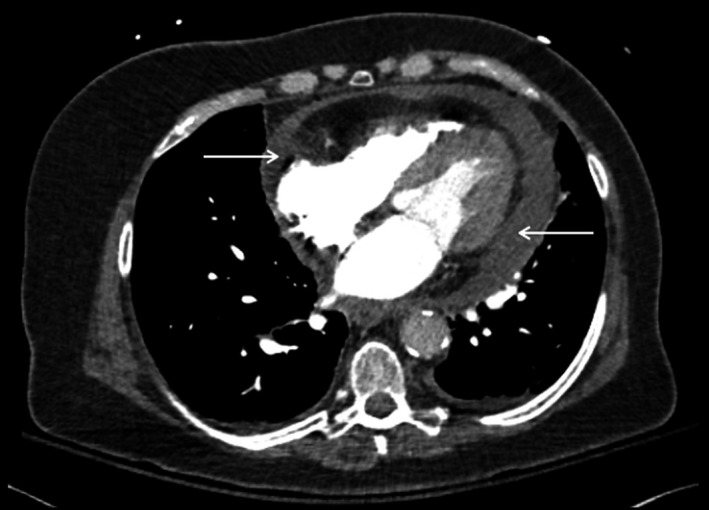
Representative axial image of a contrast‐enhanced CT of the chest, demonstrating a moderately sized moderate‐density pericardial effusion
